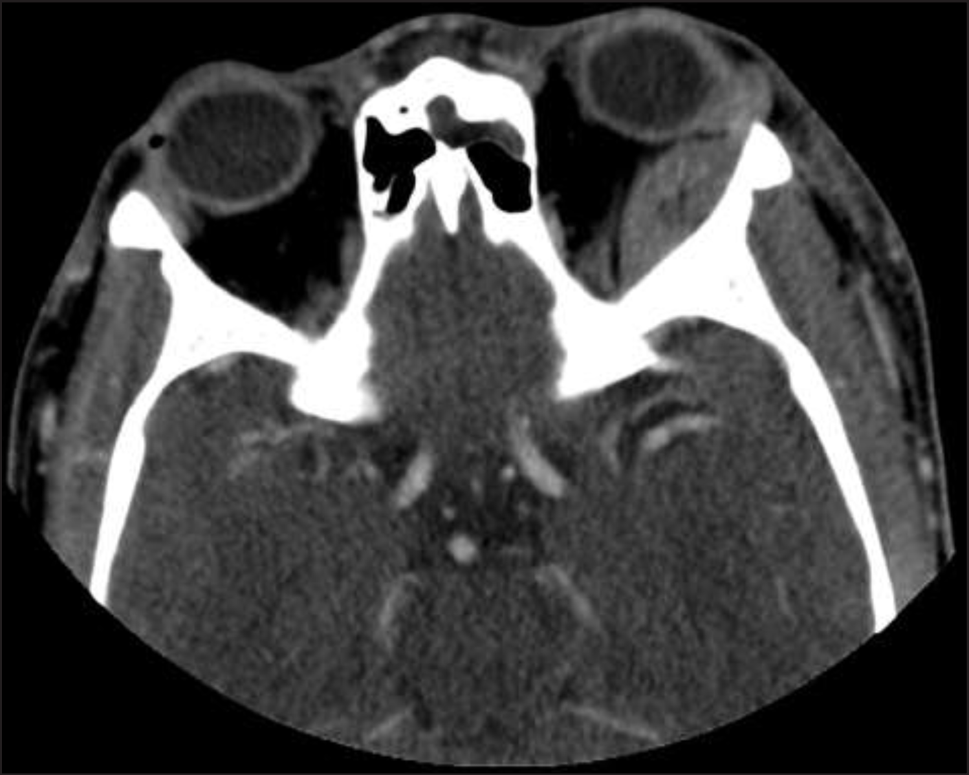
Axial contrast-enhanced CT: A biconcave lesion lining the superolateral left orbital wall.
